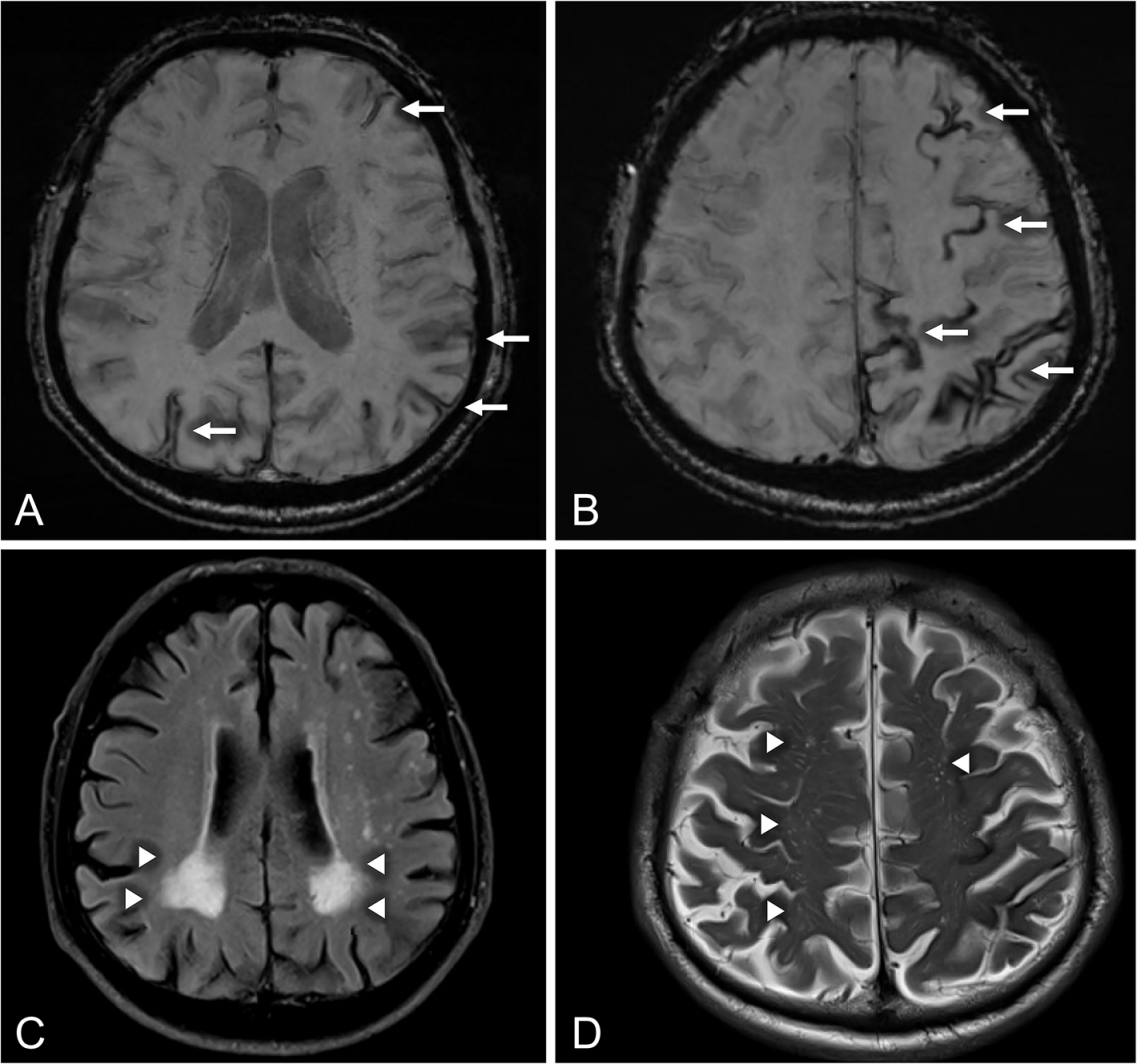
Neuroimaging findings in a patient with transient focal neurological episodes. Legend: Axial brain MRI with susceptibility-weighted imaging sequence showing rims of low signal coating the sulci of both cerebral hemispheres with left predominance, suggestive of cortical superficial siderosis (Panel a-b, arrows). Axial brain MRI with fluid-attenuated inversion recovery sequence showing symmetrical periventricular white matter hyperintensities with occipital predominance of Fazekas grade 3 (Panel c, arrowheads). Axial brain MRI with T2-weighted imaging showing enlarged perivascular spaces in centrum semiovale (Panel d, arrowheads)
